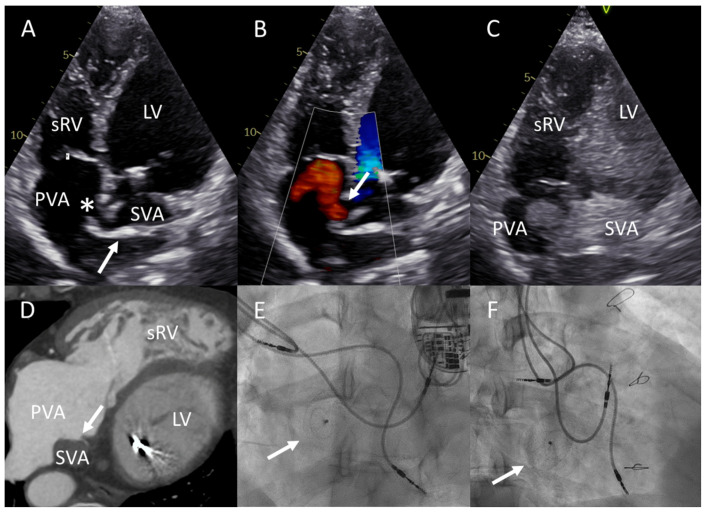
Baffle leak visualization and closure in patient B. (A) Apical four chamber view shows that the atrial baffle (arrow) directs systemic venous return atrium (SVA) to the subpulmonary left ventricle (LV) and the pulmonary venous return atrium (PVA) to the systemic right ventricle (sRV). The baffle leak is indicated by an asterisk (*). (B) Color Doppler apical four chamber view demonstrates SVA to PVA shunting through the baffle leak. (C) Significant interatrial shunting confirmed by agitated saline contrast echocardiography. Note the contrast opacification of the SVA and subpulmonary LV, and the jet crossing the baffle leak into the PVA. (D) Axial slice through a computed tomography scan at the level of the atria shows the baffle leak (arrow). (E,F) Left anterior oblique and right superior oblique fluoroscopy projections, respectively show the Occlutech Figulla Flex II Atrial Septal Defect (ASD) Occluder (arrow) projecting over the interatrial baffle. Note the presence of one ventricular (LV) and two atrial (SVA) pacemaker leads.
